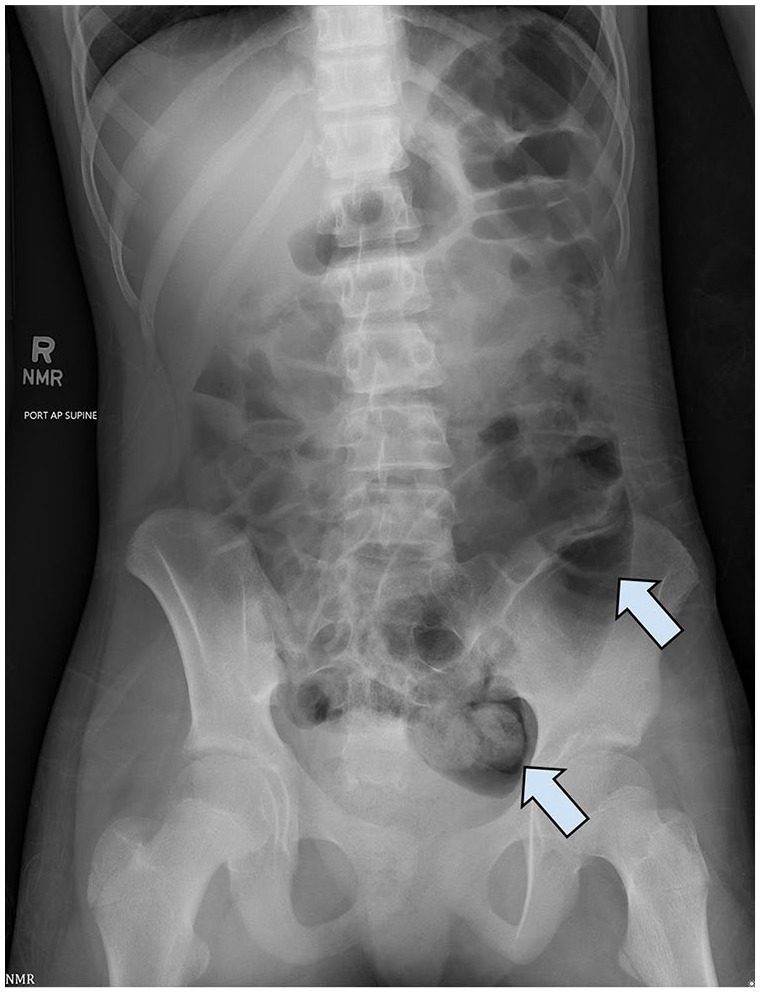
Abdominal X-ray showing dilated bowel loops (right arrow) with hyperechoic material in the distal left colon (left arrow).
